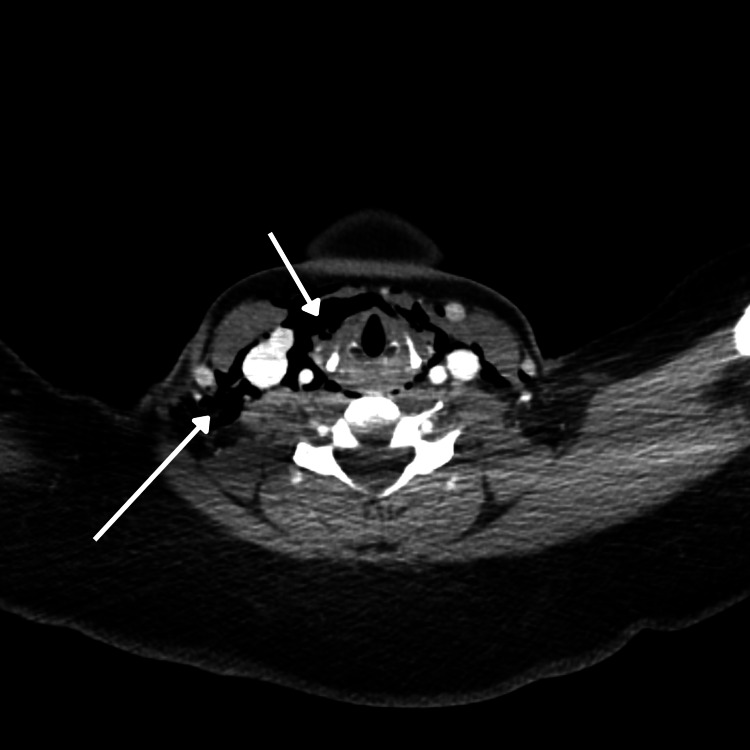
Axial contrast-enhanced CT of the thorax Pneumomediastinum, with gas (arrow) surrounding the trachea and the mediastinal great vessels, alongside anterior chest-wall subcutaneous emphysema. CT: computed tomography
